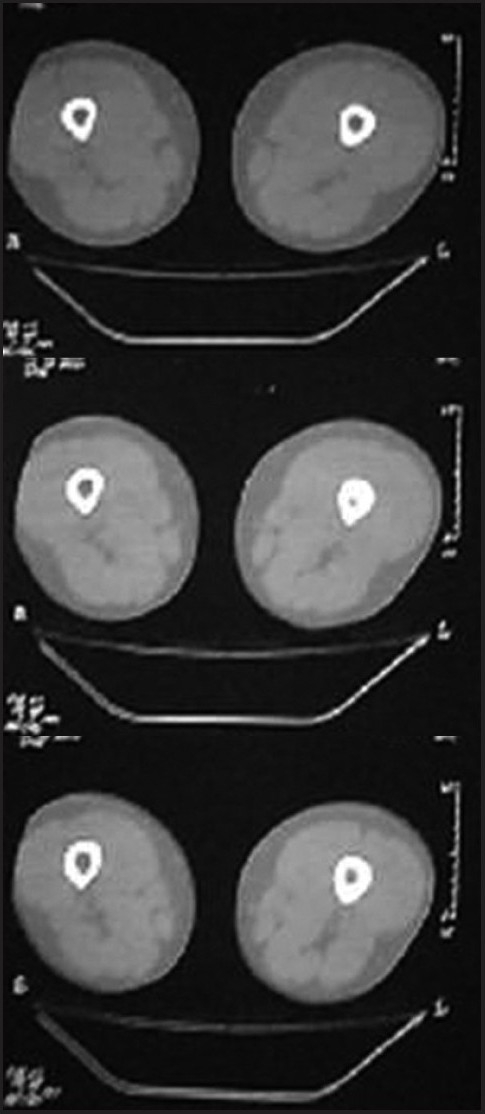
CT of the thigh outlines sclerosis, but not the nidus
